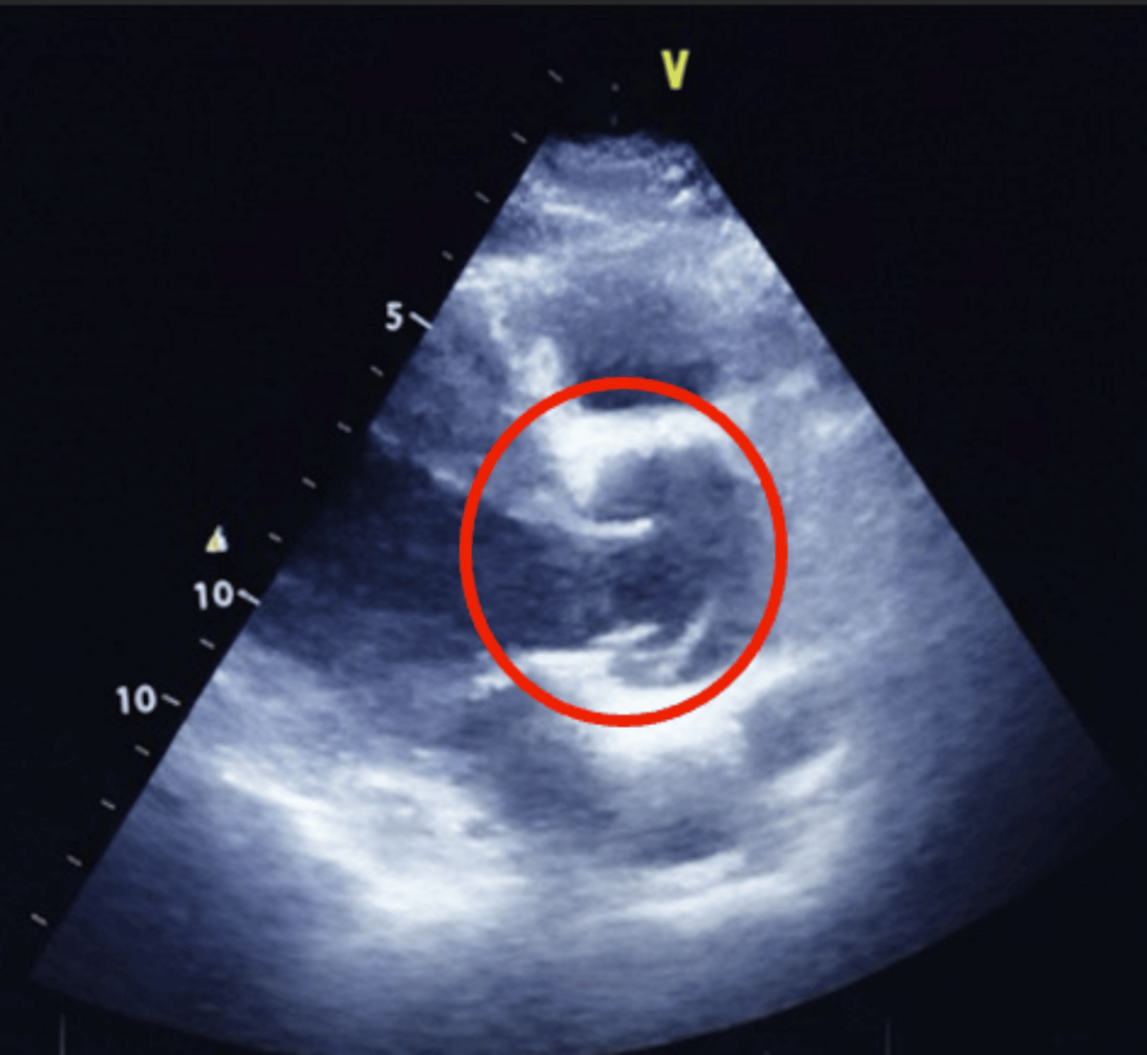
Two-dimensional parasternal long-axis view on transthoracic echocardiogram showing the double aortic valve sign (red circle)
